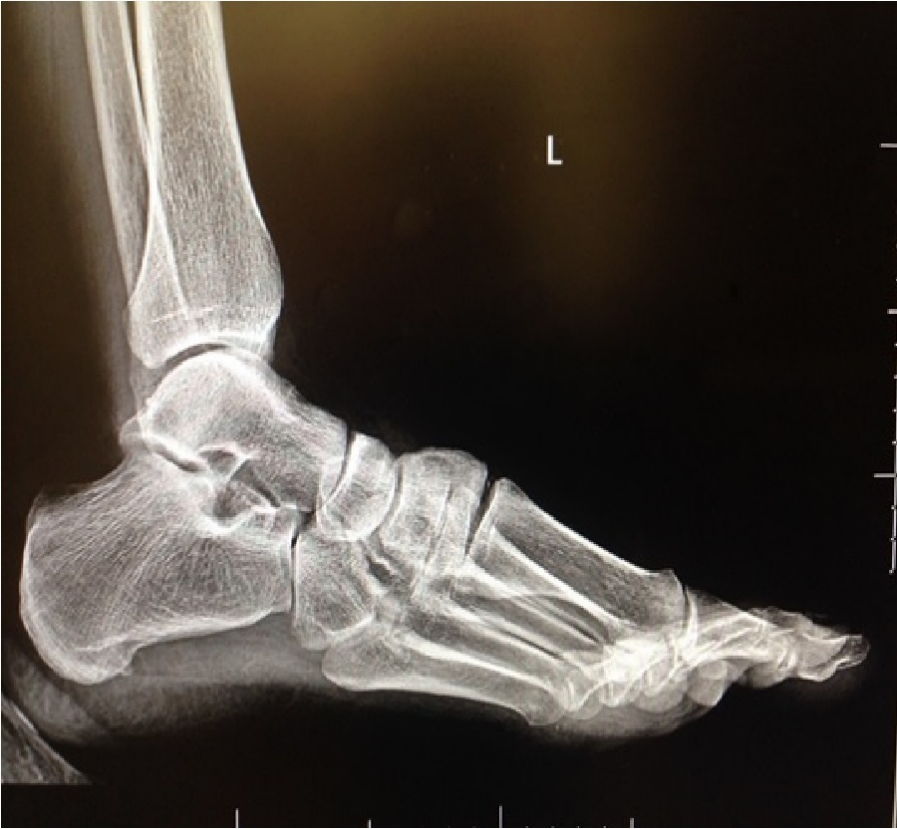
Preoperative gravity stress lateral radiograph of the left foot demonstrating widening of the first metatarsal cuneiform joint due to dorsal capsular disruption
